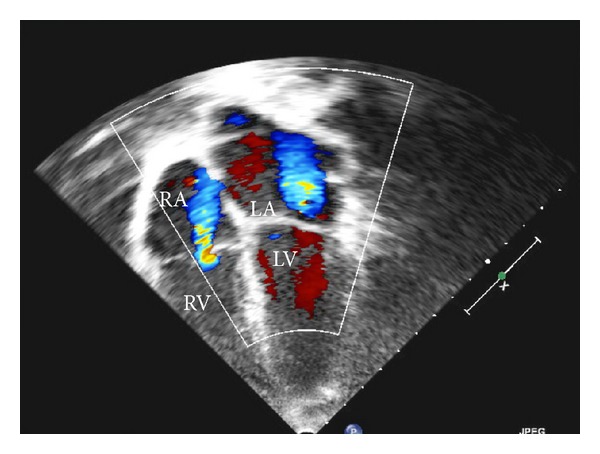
Apical 4-chamber view showing mitral valve (MV) and tricuspid valve (TV) regurgitations (demonstrated by the blue jets of retrograde flow on the Doppler study). Aortic and pulmonary valve regurgitations are not seen on this image.
